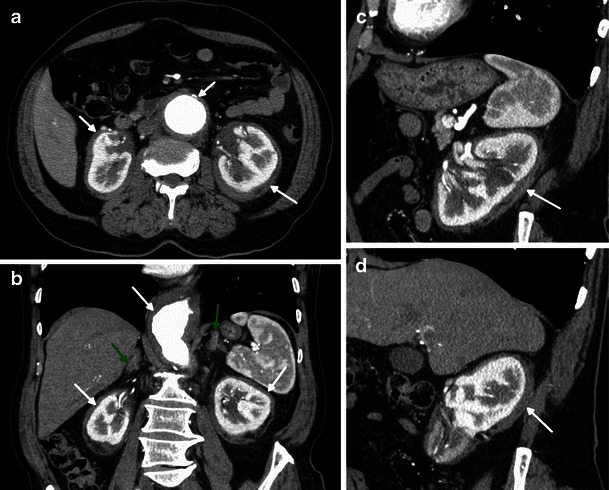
Reformatted axial (a), coronal (b) and sagittal (c, d) enhanced CT images in a 73-year-old female showing bilateral and symmetric perirenal infiltration (arrow) and bilateral adrenal thickening (green arrow). Note also the periaortic concentric soft tissue infiltration (arrow) and aortic aneurysm
